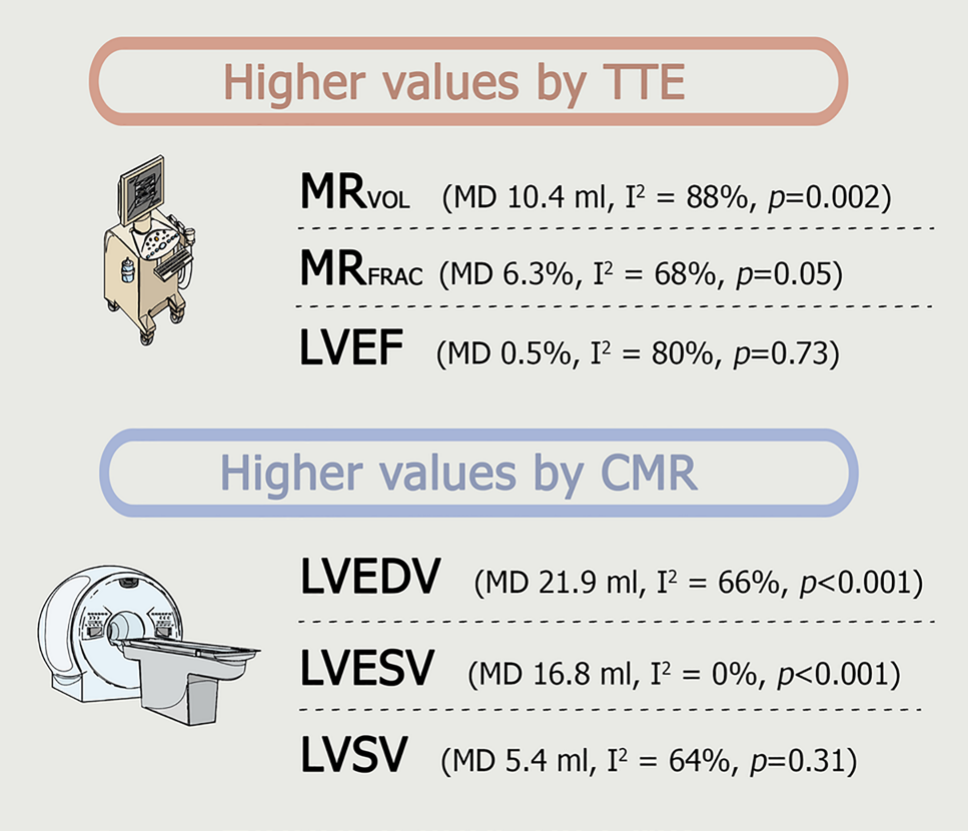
Summary of the meta-analyses mean difference (MD) results. Heterogeneity (I^2^) ≥ 50% is considered high. p-value ≤ 0.05 marks a significant difference between the included studies per variable. *CMR* cardiac magnetic resonance, *LVEF* left ventricular ejection fraction, *LVEDV* left ventricular end-diastolic volume, *LVESV* left ventricular end-systolic volume, *LVSV* left ventricular stroke volume, *MR*_*FRAC*_ mitral regurgitation fraction, *MR*_*VOL*_ mitral regurgitation volume, *TTE* transthoracic echocardiography
